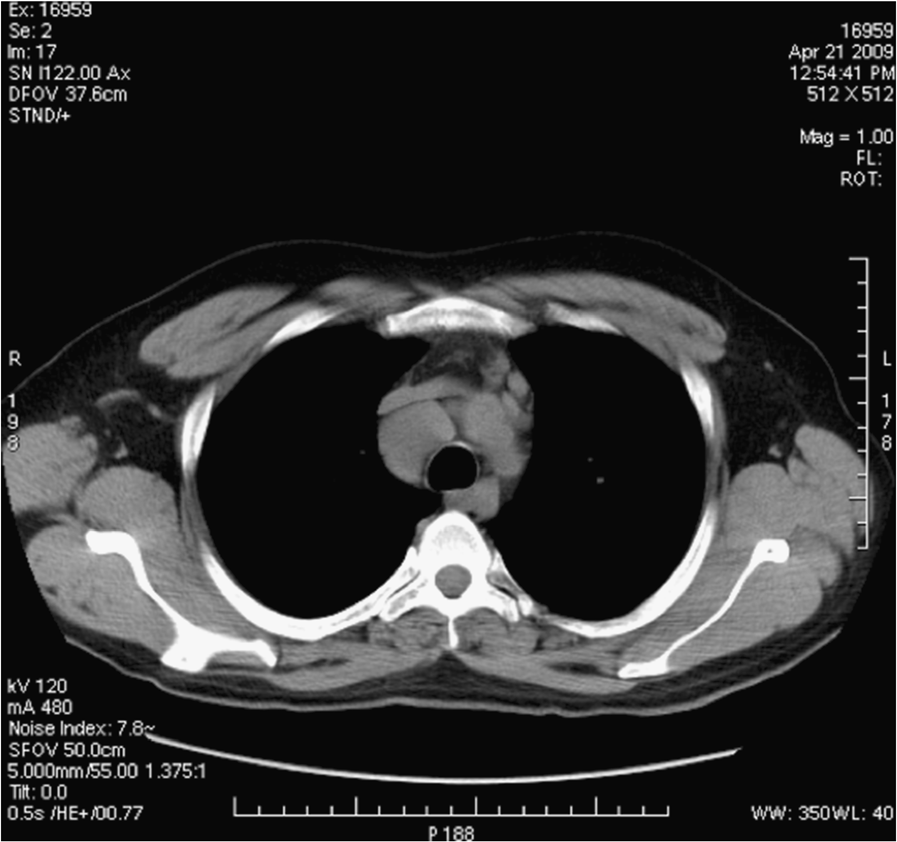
High resolution computed tomography of the thorax showing mediastinal lymphadenopathy in a patient with grade 2 sarcoid with respiratory symptoms.
